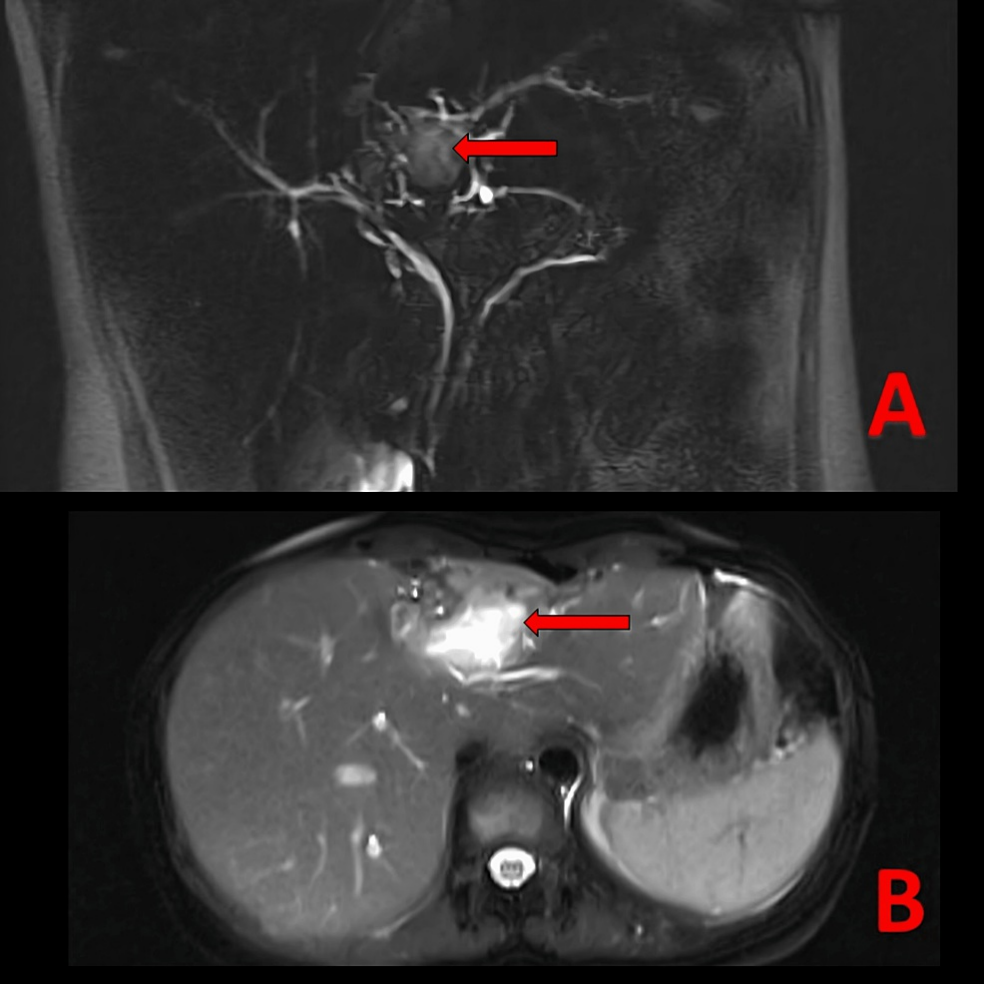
T2-weighted magnetic resonance imaging (MRI) post endoscopic retrograde cholangiopancreatography (ERCP) stenting (A) Coronal plane and (B) Axial plane, showing one of the biliary radicals of the left lobe reaching up to the posterior-superior wall of the residual hydatid cyst with suspicious communication.
